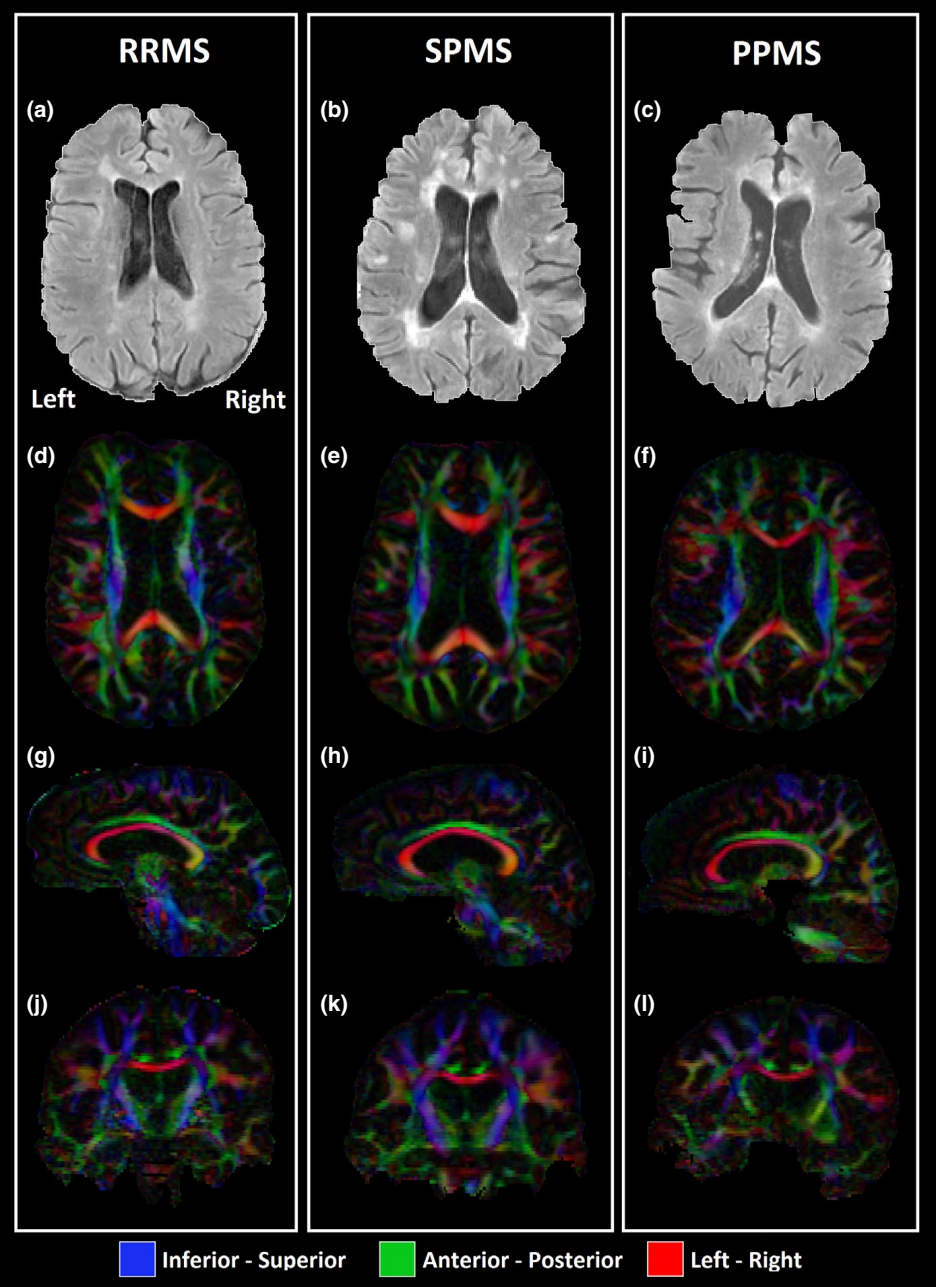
Axial FLAIR images showing periventricular lesions, and axial, sagittal, and coronal views of the 1.7 mm isotropic first eigenvector fractional anisotropy (FEFA) maps in a RRMS (a, d, g, j, 51 years old, male, lesion number = 24, total lesion volume = 8.8 cm^3^, time since diagnosis = 14 years, Expanded Disability Status Scale [EDSS] = 6.0), a SPMS (b, e, h, k, 52 years old, female, lesion number = 17, total lesion volume = 19.1 cm^3^, time since diagnosis = 24 years, EDSS = 6.5), and a PPMS patient (c, f, i, l, 54 years old, male, lesion number = 21, total lesion volume = 15.5 cm^3^, time since diagnosis = 3 years, EDSS = 6.5). Red—left/right, green—anterior/posterior, blue—inferior/superior
